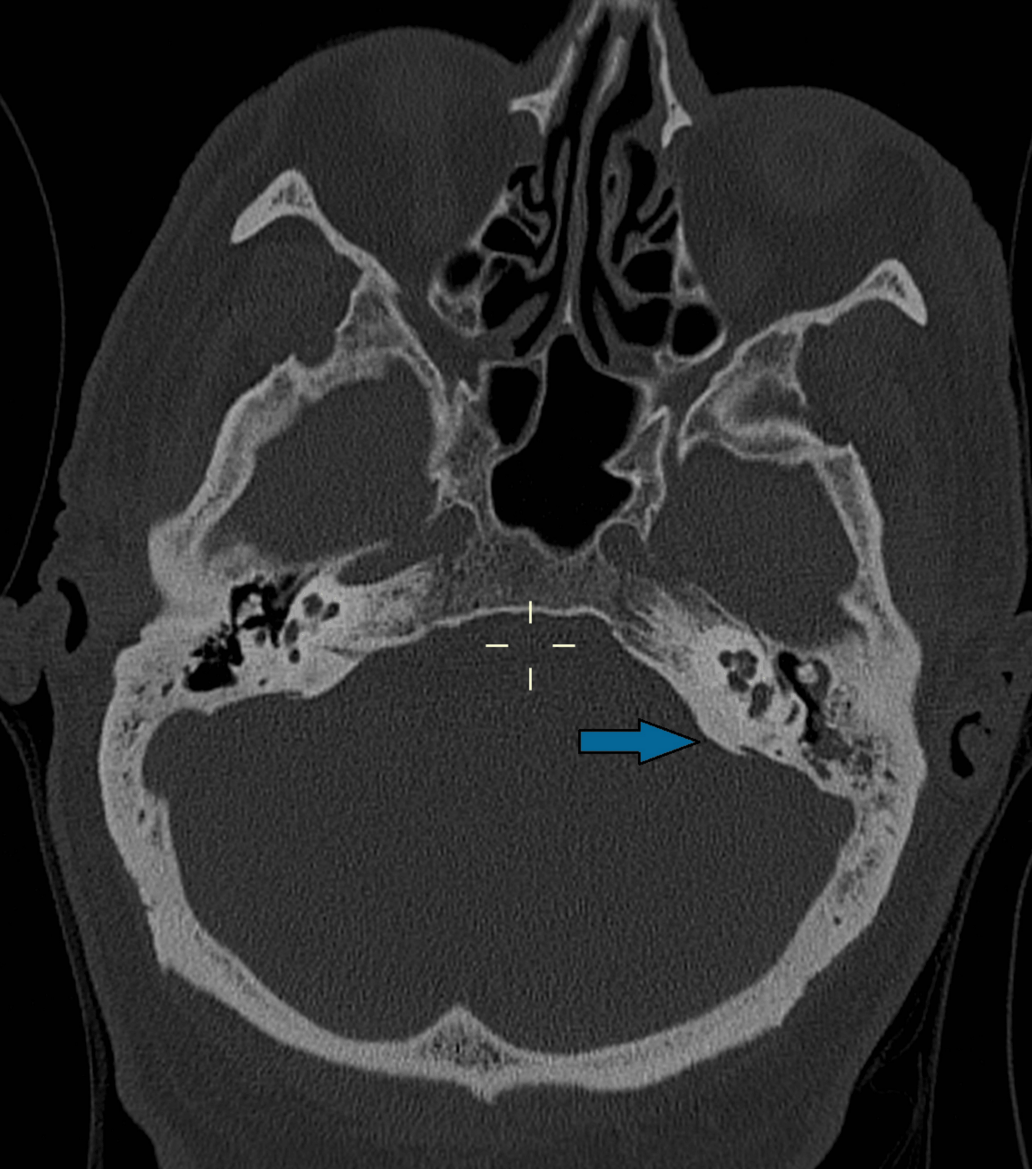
CT scan showing left otomastoiditis.
